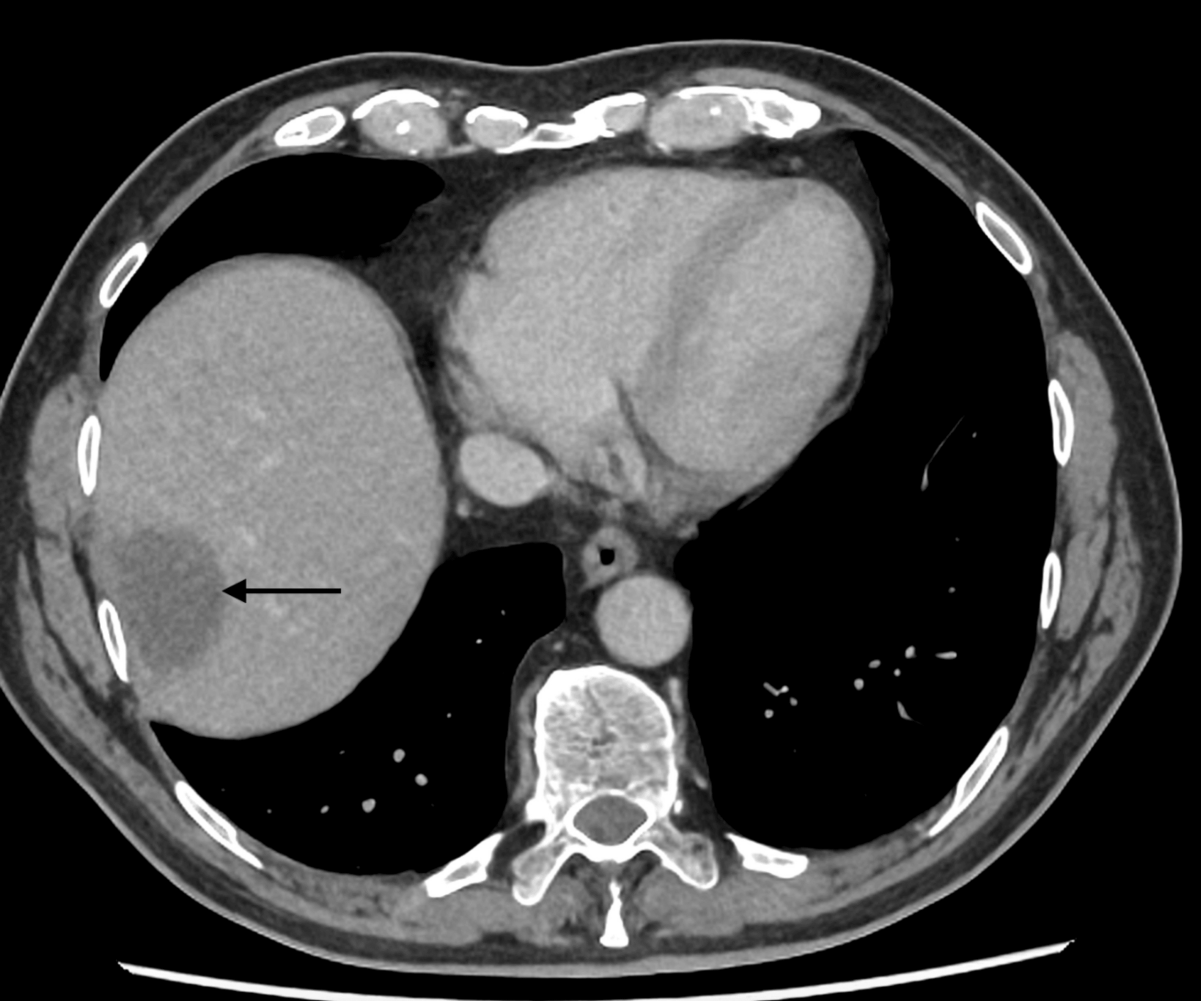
CT of the abdomen showing a hypoattenuating lesion within the right lobe of the liver in keeping with a hepatic abscess.
